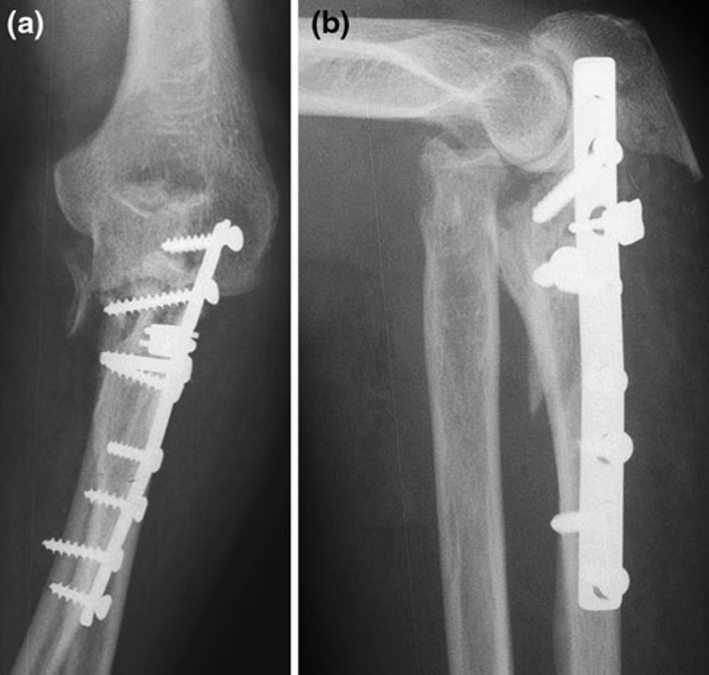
AP and lateral radiograph showing a nonunion after plate fixation of a Monteggia fracture. The plate is not on the tension (=dorsal) side but rather on the medial side. The apex posterior deformity recurred with subluxation of the radial head
